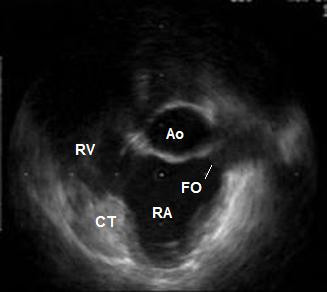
ICE during atrial systole before the jump of the transseptal needle into the oval fossa. The LA cavity is virtually missing at this time point of the cardiac cycle. Ao – non-coronary sinus of the aorta; CT – terminal crest; FO – oval fossa; RA – right atrium; RV – right ventricle.
